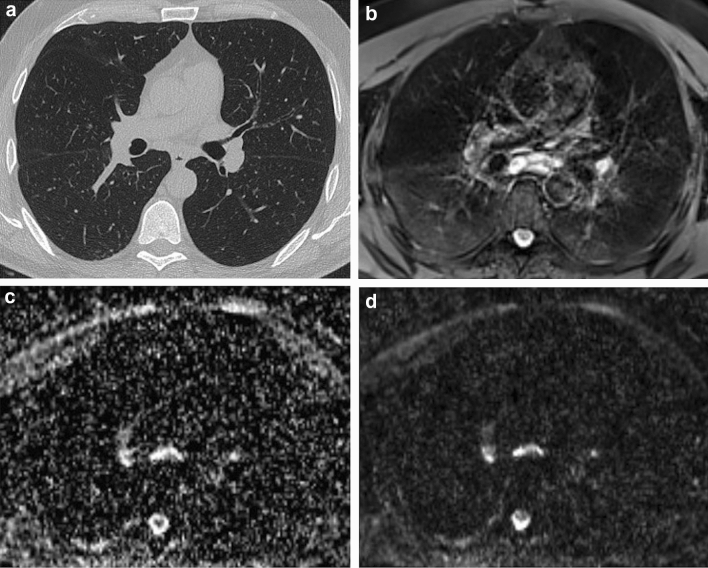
Ground-glass opacity with negative CT. 46-year-old male. a End-inspiratory axial CT after 1 month from discharge, b Free-breathing PD-weighted axial image, c Apparent Diffusion Coefficient Map, and d diffusion-weighted imaging (DWI) free breathing (*b* = 1000 s/mm^2^). Note areas of GGO on both the right and the left lower lobes visible on the PD-weighted image not corresponding to any alteration on the CT scan. No restricted diffusion on DWI nor hyperintensity on ADC map depicted
